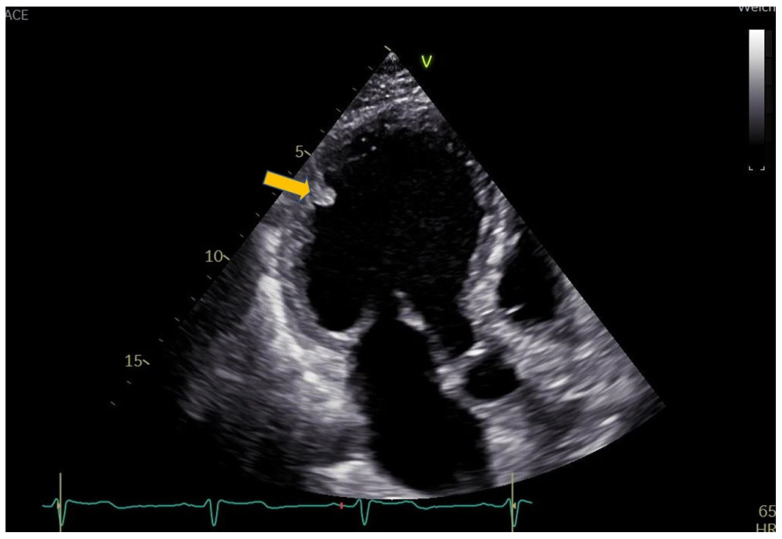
Day 9. Transthoracic echocardiography, apical three-chamber view. Marked reduction in the size of the left ventricular thrombus.
